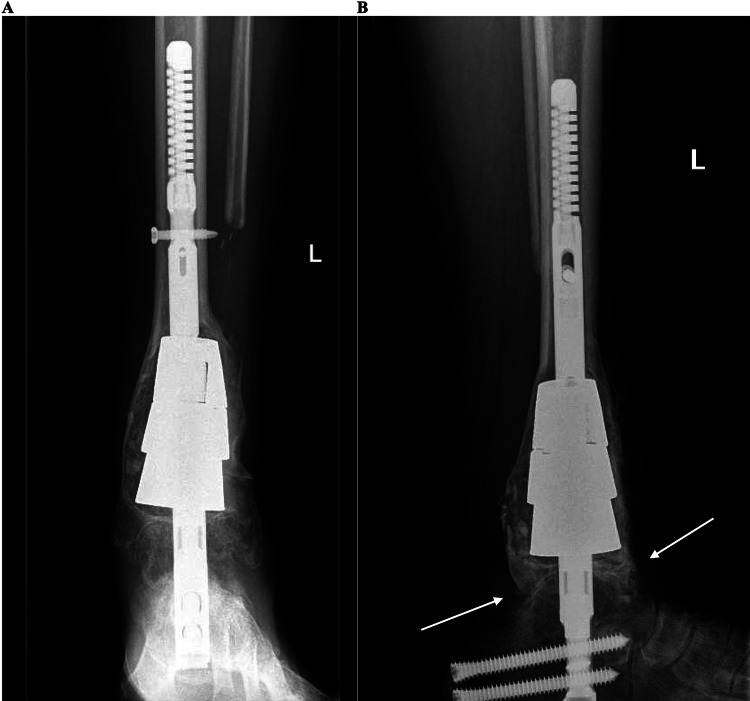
Postoperative x-rays AP (A) and lateral (B) x-ray views of the left ankle with trabecular metal cones and tibiotalocalcaneal nail in place six months postoperatively. The tibiotalar fusion site is marked with arrows in (B).
